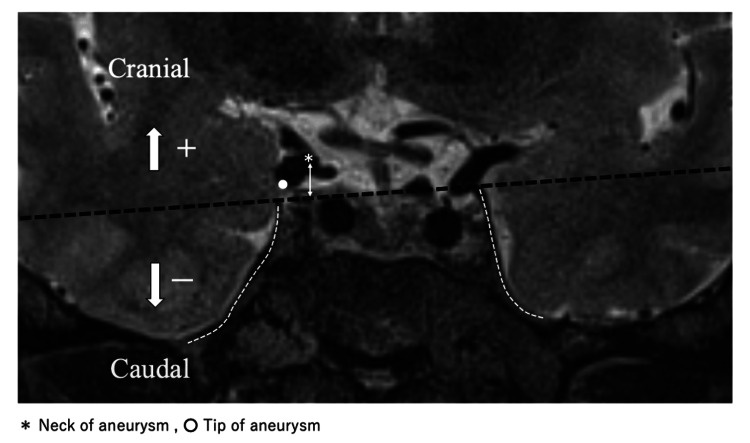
Measurement of the distance from the tentorium to the aneurysmal neck or tip A coronal image of the preoperative T2-weighted 3D image is shown. The bilateral tentorium is indicated with a white dot-dash line. A line is drawn connecting both bilateral tips of the tentorium (black dot-dash line). Aneurysmal projections are classified into two types: superior to tentorium (superior type) and inferior to tentorium (inferior type). If they are located X mm cranial to the line, the distance is identified as + X mm. If they are located Y mm caudal to the line, the distance is identified as - Y mm. An asterisk indicates the aneurysmal neck. A white dot indicates the tip of the aneurysm. A white double arrow shows the distance between the tentorium and the aneurysmal neck.
